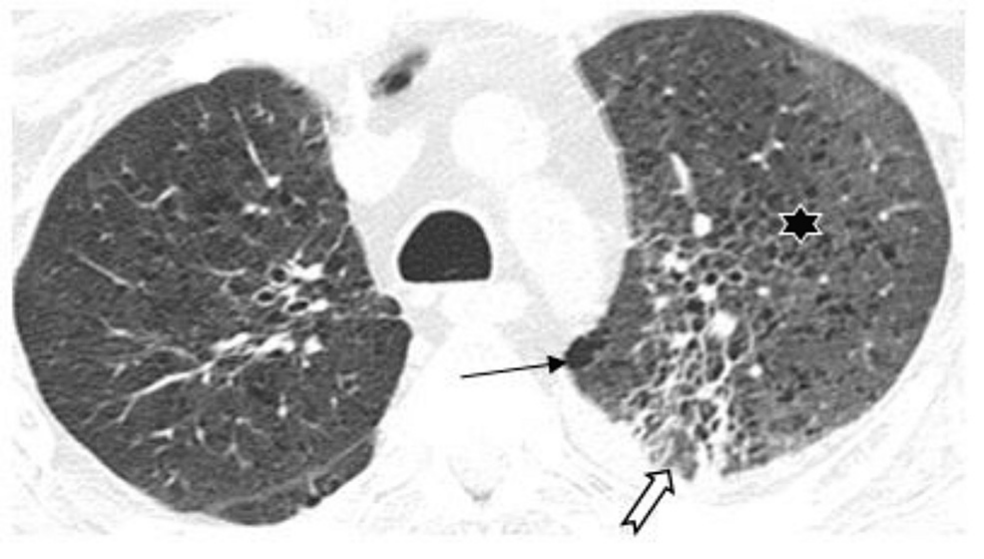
HRCT thorax axial section of 70Y/M patients with CO-RADS 5 showing centrilobular (star) and paraseptal (arrow) emphysematous changes in left upper lobe. Few parenchymal bands (block arrow) noted in apicoposterior segment of left upper lobe.
